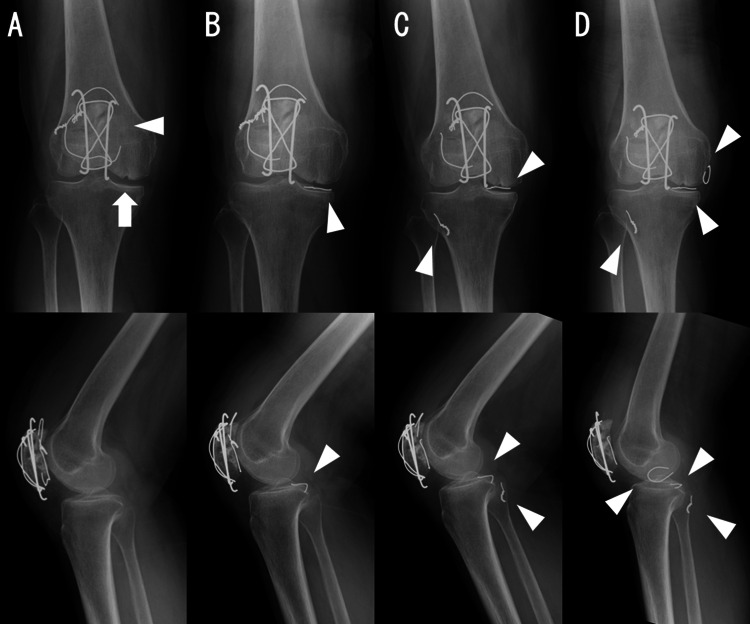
Sequential radiographs showing wire breakage and migration A: Radiographs at 12 months postoperatively (AP and lateral views). The patellar non-union and breakage of the circumferential wire are visible (arrowhead). Note the subchondral bone depression on the medial femoral condyle (arrow). B-D: Serial radiographs showing the progressive migration of wire fragments at 15 months (B), 16 months (C), and 17 months (D) postoperatively (arrowhead). AP, anteroposterior.
